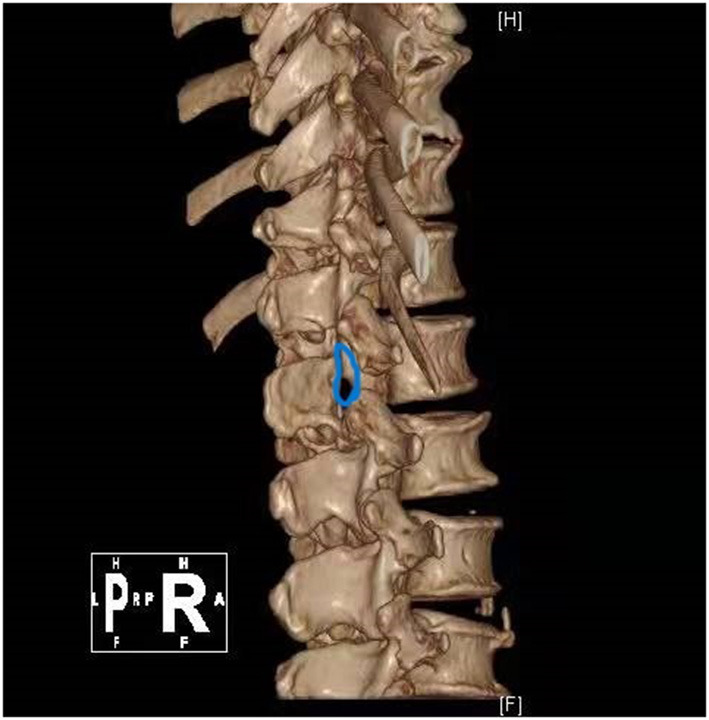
Postoperative 3D CT reconstruction of the case 1.
